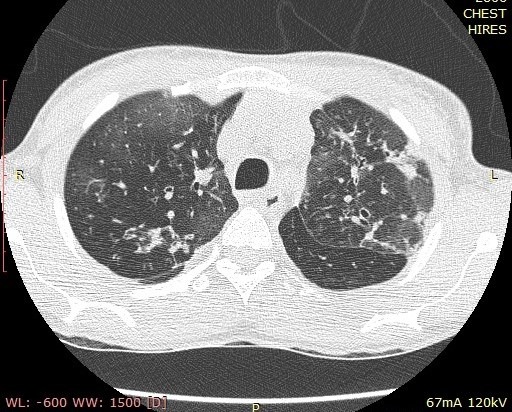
Chest CT scan showing Signet ring appearance in both lung fields in the upper lobes
